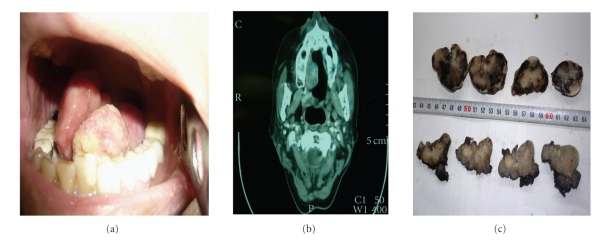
Macroscopic features and radiological imaging of SpCC. (a) Photographic view of the tumor just before operation. (b) Polypoid tumor mass in oral cavity on CT imaging. (c) The cut surfaces of the tumor.
